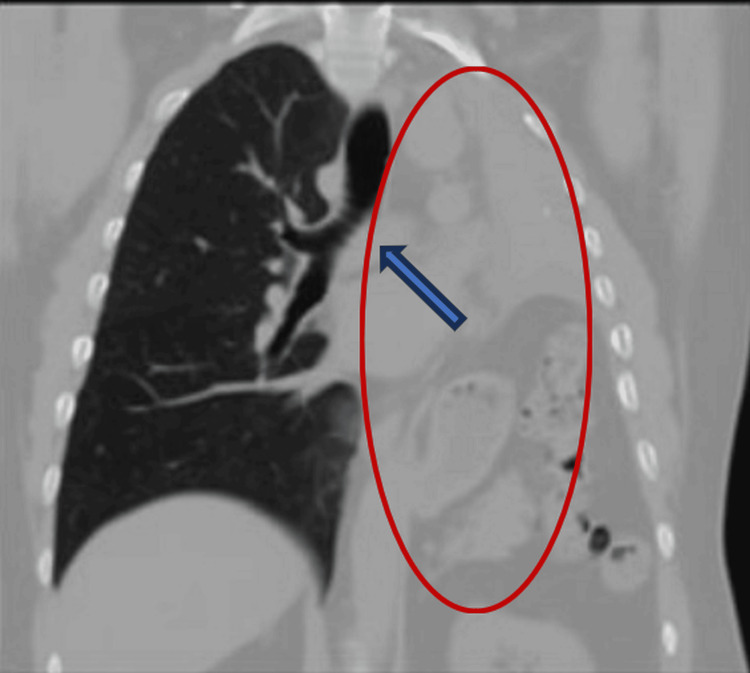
Preoperative coronal chest computed tomography Complete obstruction of the left main bronchus (blue arrow). The red circle outlines the expected anatomical location of the left lung, which is nearly completely collapsed secondary to chronic left main bronchus obstruction, resulting in functional single-lung physiology. The right lung remained fully expanded and served as the patient's only effective gas-exchanging lung during the procedure. These anatomical findings correlated with preoperative spirometry demonstrating severe restrictive ventilatory impairment.
